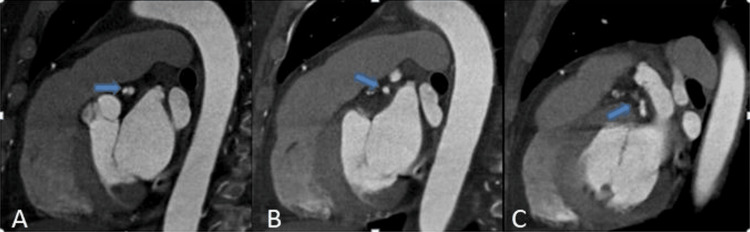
CTCA showing a large calcified mixed density plaque/thrombus in proximal (A), mid (B), and distal (C) parts of LAD causing >90% thrombosis (blue arrows) CTCA: computed tomography coronary angiography; LAD: left anterior descending artery
